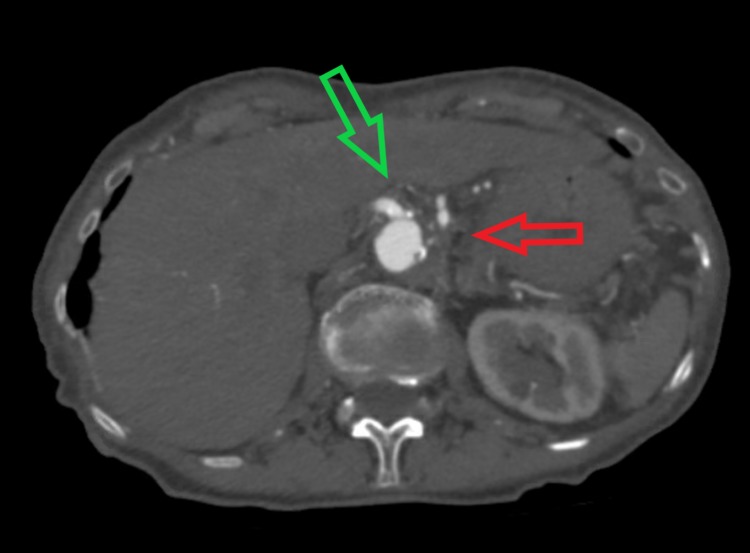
Computed tomography angiography (CTA) of abdomen/pelvis shows occlusion of the proximal superior mesenteric artery (red arrow) and celiac artery (green arrow)
